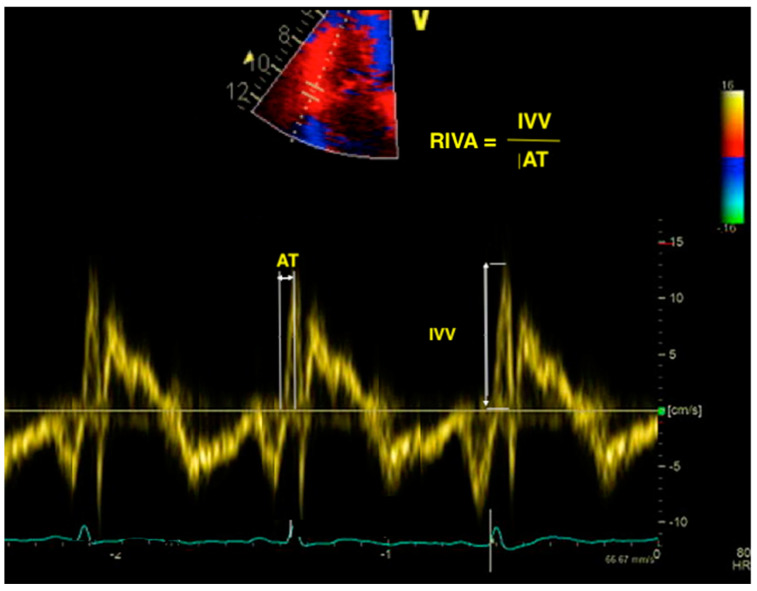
Calculation of RIVA using TDI. RIVA—right isovolumic acceleration; TDI—tissue Doppler imaging; IVV—isovolumic velocity; AT—acceleration time.
